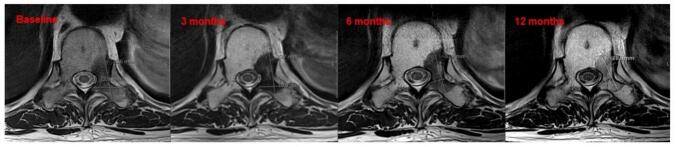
Baseline and serial T2-weighted MRI images obtained at 3, 6, and 12 months following SBRT to a T10 metastasis demonstrate marked post-treatment signal alterations.
